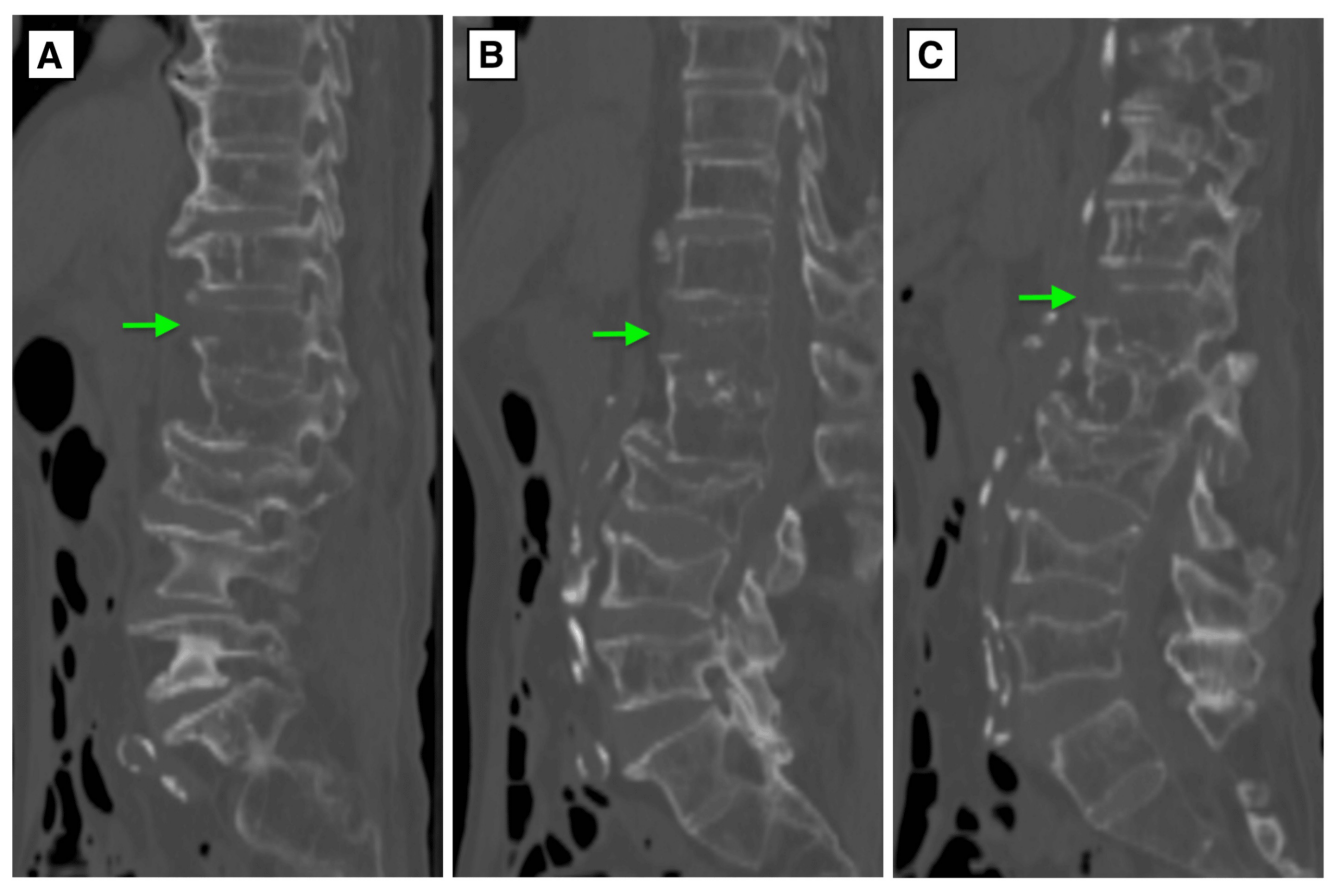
Sagittal CT images of the first lumbar vertebra (Case 1). (A) Right pedicle level. The arrow indicates the fracture site. (B) Midline. The arrow indicates the fracture site. (C) Left pedicle level. The arrow indicates the fracture site.
